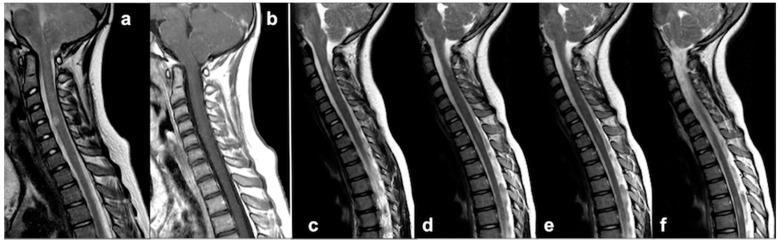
Single and multiple spinal unidentified bright objects (UBOs) in two different NF1 patients. Patient 1: Sagittal TSE T2w (a) and contrast-enhanced TSE T1w MRI (b) of a 11 year-old boy, showing an isolated and well-defined T2-hyperintense, T1-hypointense, unenhancing lesion localized in the ventral portion of the cervical spine (C4-C5 level). Patient 2: Sagittal TSE T2w at the three most significant levels (c–f) of a 4 year-old girl, showing multiple spinal lesions disseminated through cervical and thoracic spinal cord.
